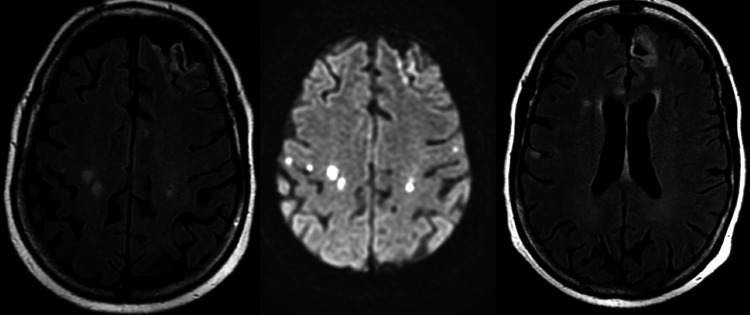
Non-contrast MRI 10/31/2022 Left: FLAIR MRI showing new acute areas of cerebral ischemia most predominantly in the centrum semiovale of both of the cerebral hemispheres Middle: DWI MRI showing corresponding areas of ischemia of bilateral centrum semiovale Right: FLAIR MRI demonstrating old infarct (10/28/2022) with encephalomalacia in the anterior left frontal lobe
